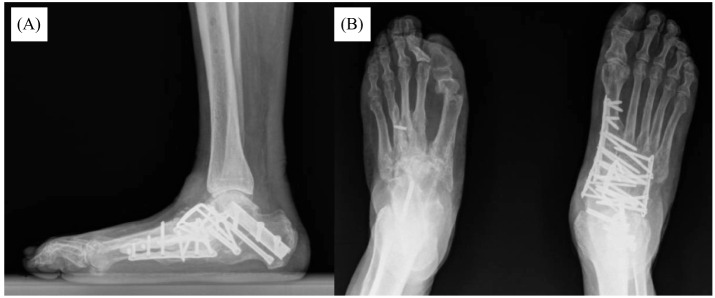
Most recent radiographs at final follow-up. (A) Lateral radiograph. (B) Bilateral anterior-posterior radiograph, revealing restoration of the arch of the foot, through the use of multiple osteotomy sites and internal fixation with a variety of plate, screw, and beam constructs.
